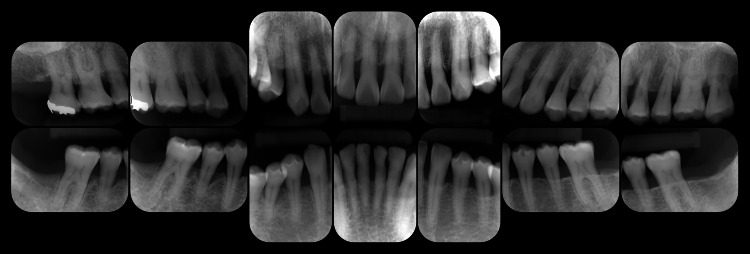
Radiographic view at two years of SPT SPT: supportive periodontal therapy
